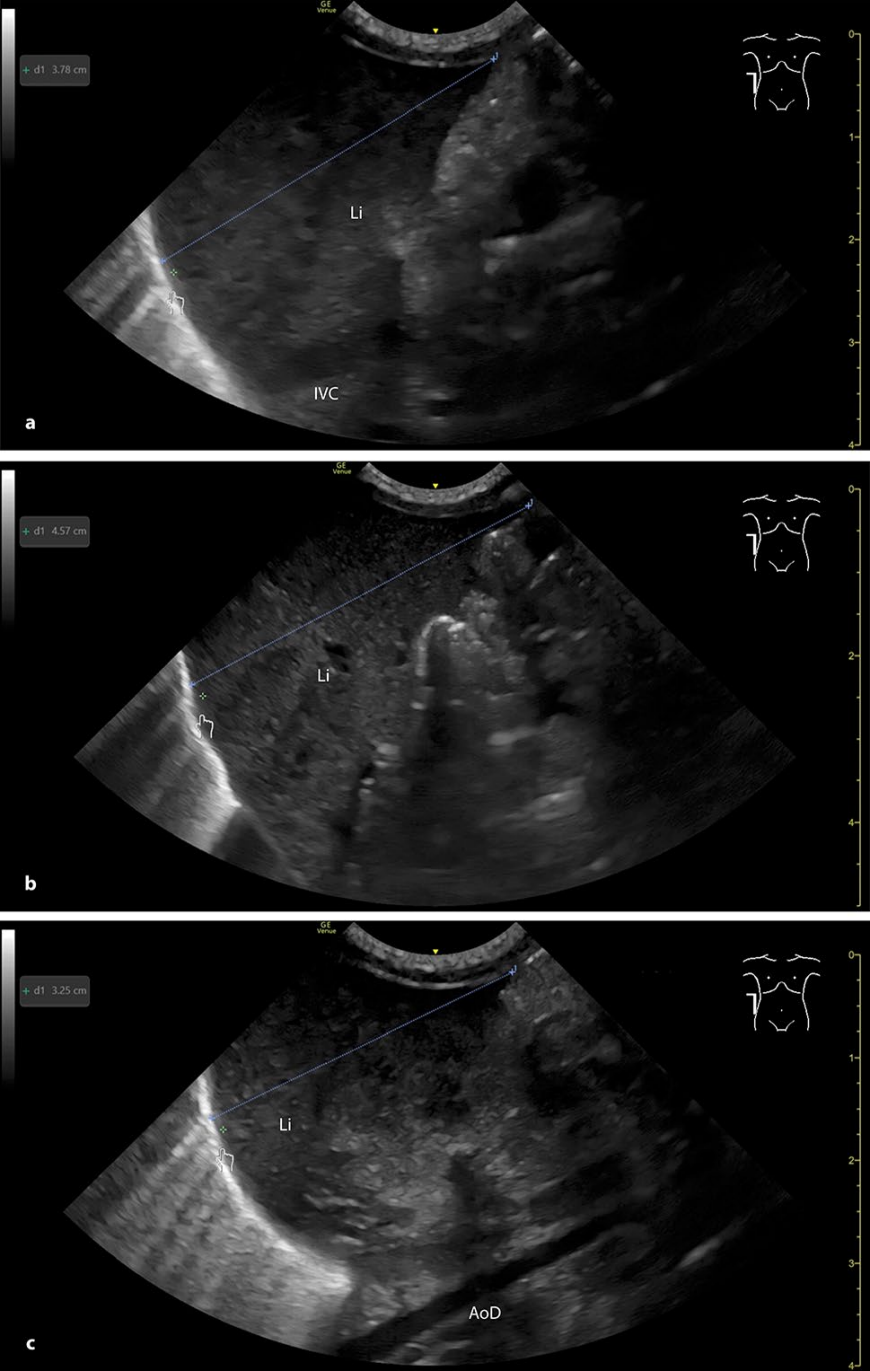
Sonographic craniocaudal liver length measurement in the right AAL in the lateral plane. a the probe is held parallel to the mattress (correct technique). b the probe is tilted cranially. c the probe is tilted caudally. AAL: anterior axillary line, AoD: descending aorta, IVC: inferior vena cava, Li: liver
